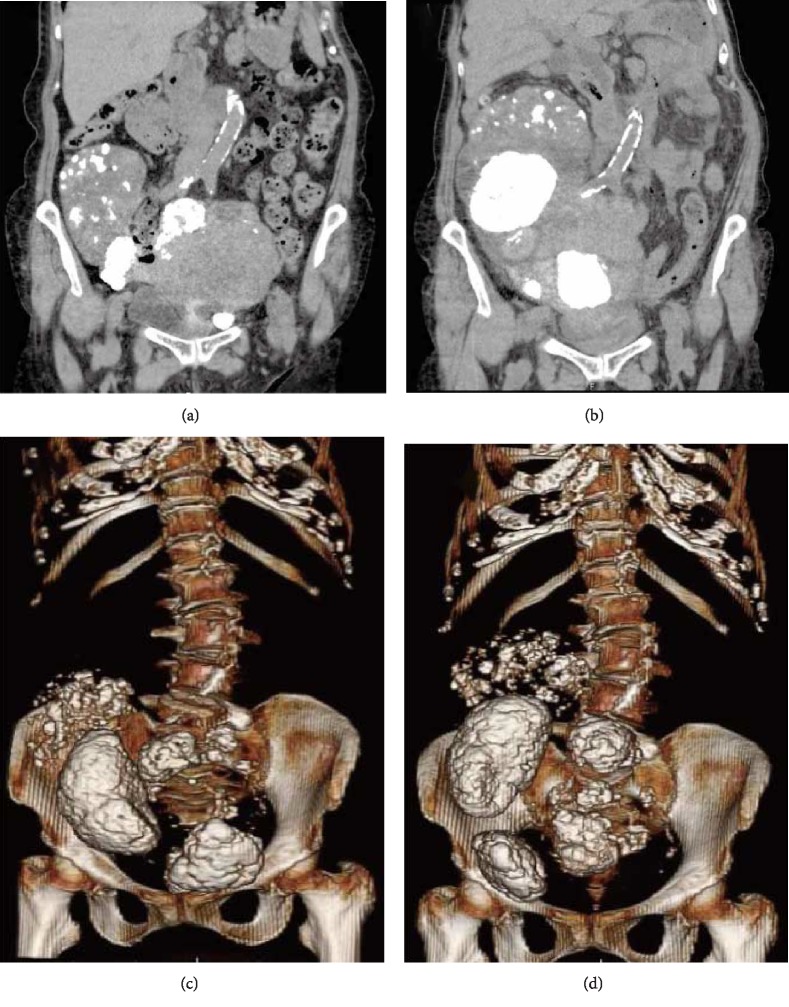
Computed tomography (CT) findings of the myomatous uterus. (a) Coronal section of the abdomen one year before the uterine torsion. The myomatous uterus and calcified portion are observed. (b) Coronal section of the abdomen at the time of uterine torsion. The myomatous uterus significantly increased in size, and the calcified lesion was displaced. (c) Three-dimensional reconstruction images of the lower abdomen one year before the uterine torsion. (d) Three-dimensional reconstruction images of the lower abdomen at the time of uterine torsion. The calcified area inside the uterus was largely displaced.
